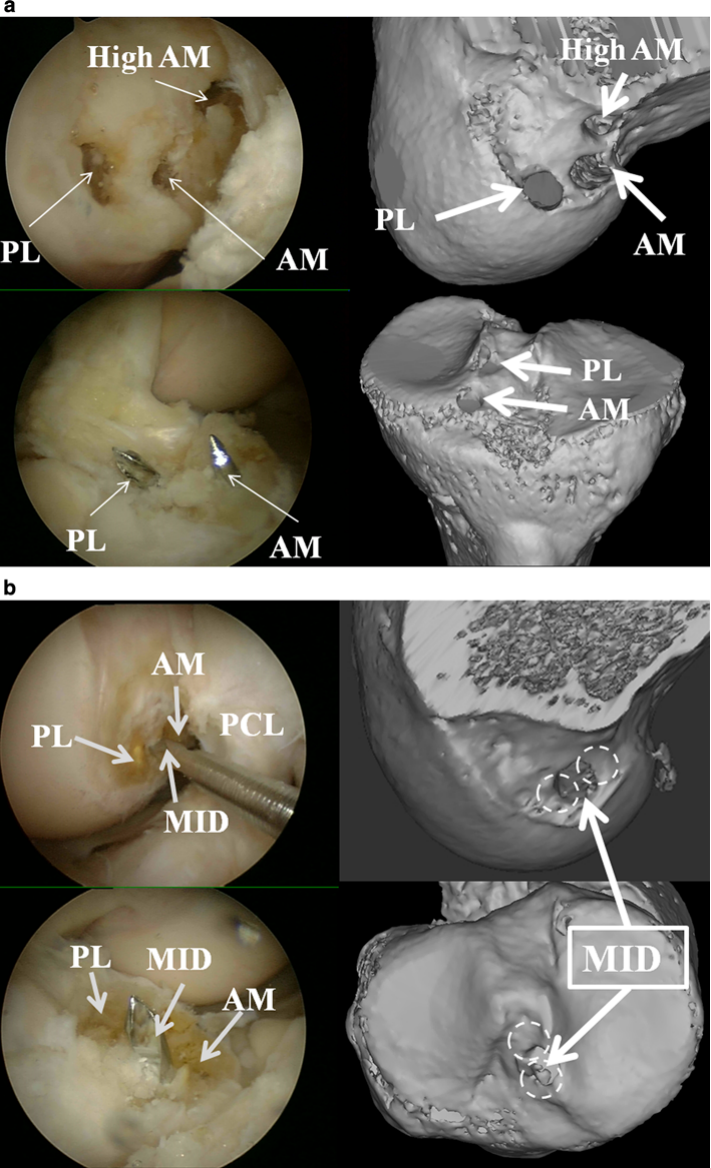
a Three femoral tunnels (PL, AM and high AM) and two tibial tunnels (PL and AM) were created in a multiple-reconstructed knee (arthroscopic view and 3D CT scan reconstruction). b For the MID–MID reconstruction, the tibial and femoral tunnels were drilled between the centre of the AM and PL footprints of the tibia and femur (arthroscopic view and 3D CT scan reconstruction)
